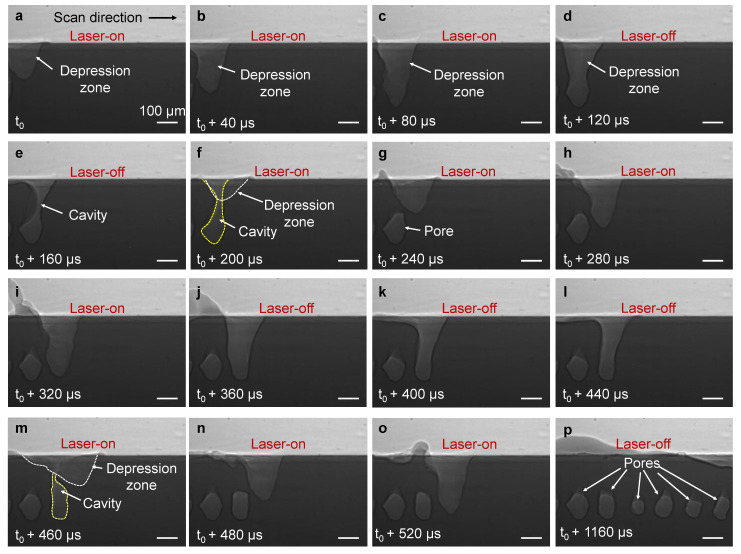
Pore pattern formation from cavities at low laser frequency: (a–p) Dynamic X-ray images showing the formation of pores from a cavity during pulsed-wave laser melting of Ti-6Al-4V at a laser frequency of 4 kHz, a duty cycle of 60%, a laser power of 470 W and a laser scan speed of 0.5 m/s. The pore formation from cavity in two consecutive pulses is shown in (a–o). Note that some image frames during the laser-on period and laser-off period have been skipped. The movie showing pore formation dynamics is available in the Supplementary Material (Movie S8).
